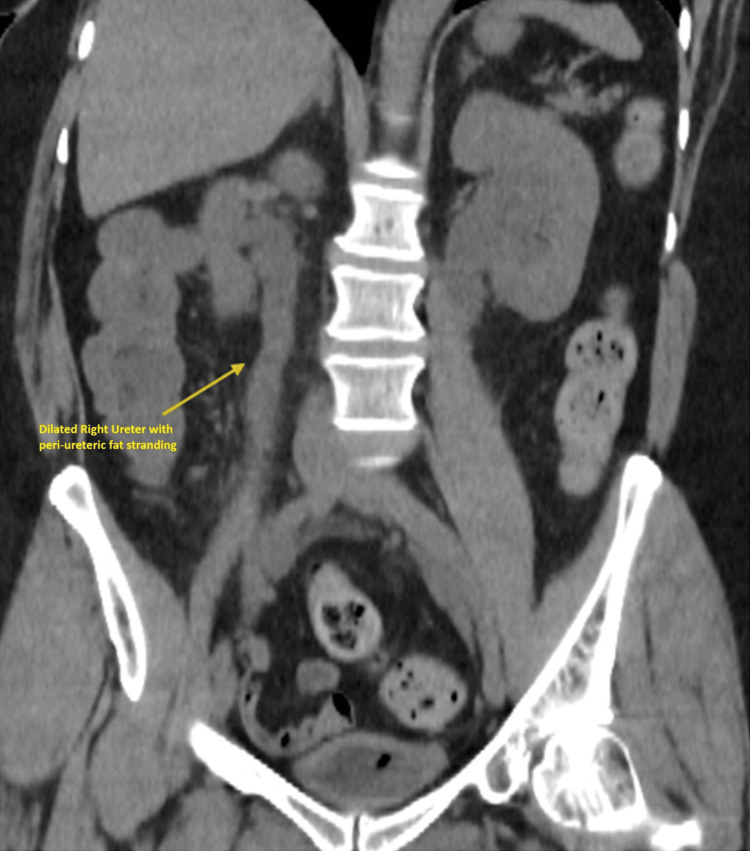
Coronal CT renal tract image showing dilatation of the right ureter with adjacent peri-ureteric inflammatory fat stranding (yellow arrow)
